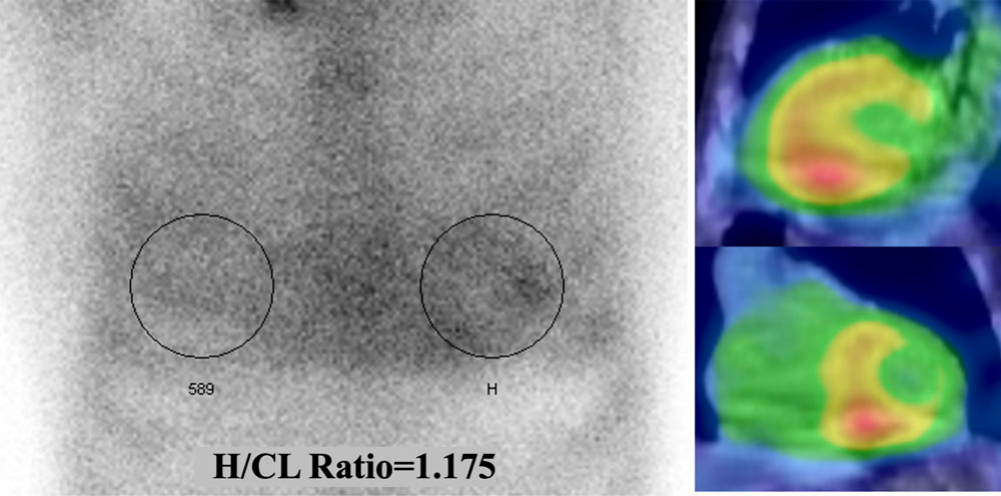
^99m^Technetium‐pyrophosphate scintigraphy showed high cardiac uptake greater than rib uptake (grade 3). The quantitative value of myocardial‐to‐contralateral lung uptake ratio at 3 h was 1.175. H/CL, myocardial‐to‐contralateral lung uptake ratio.
